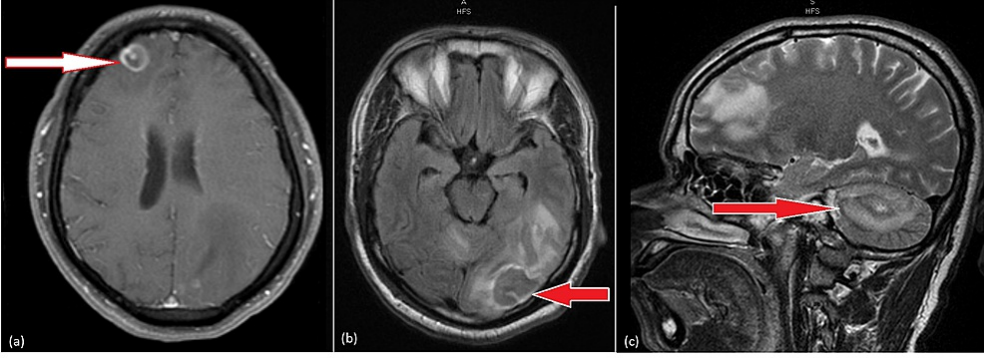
Contrast-enhanced MRI images for Case 3 Numerous ring-enhancing lesions with considerable surrounding vasogenic edema are seen in (a) the right anterior frontal lobe (axial view) measuring 1.3 x 1.3 cm with extensive surrounding edema; (b) the left occipital lobe (axial view) measuring 2.2 x 3.5 cm; (c) the right cerebellar hemisphere (sagittal view) measuring 2.2 x 2.6 cm.
